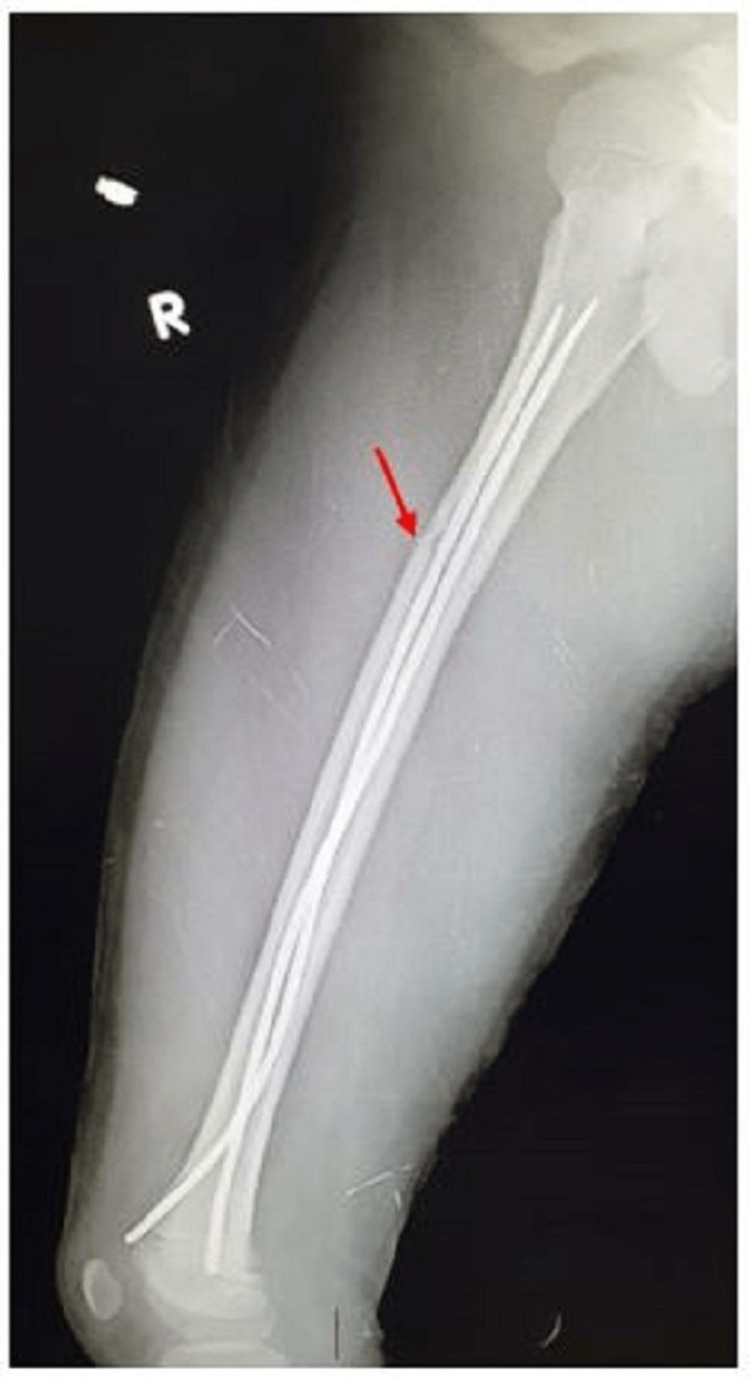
Lateral radiograph of a nine-year-old male with right subtrochanteric fracture (red arrow) who underwent TENS fixation TENS: titanium elastic nailing system
